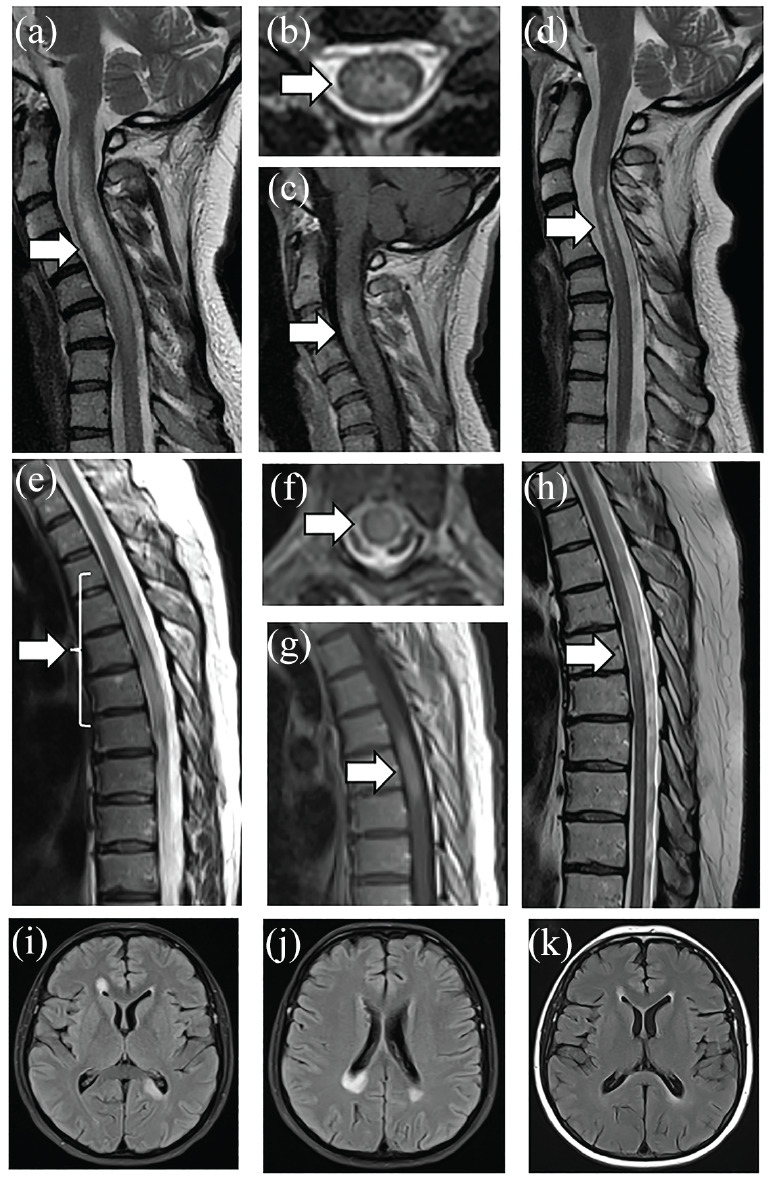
(a to d) A 41-year-old women ultimately diagnosed with AQP4-IgG NMOSD presented with neck pain and left arm weakness with rapid progression more than 2 weeks to quadriplegia and breathing difficulties. Cervical MRI showed longitudinally extensive lesion on T2-weighted imaging with cord swelling (a), central location on axial images (b), and ring-like contrast enhancement (c). The patient was treated with intravenous methylprednisolone followed by plasma exchange with improvement. Follow-up imaging 9 months later showed residual T2-weighted hyperintensity in the central portion of the cord (d). (e to k) A 45-year-old women presented with numbness and weakness in the left leg progressing more than 3 weeks to severe paraplegia with double incontinence and Th6 sensory level despite intravenous methylprednisolone. Spinal cord MRI showed a longitudinally extensive lesion spanning the cord continuously from Th3 to Th7 with mild cord swelling (e). The lesion was located centrally (f) and partially contrast-enhancing (g). Brain MRI showed large poorly demarcated periventricular lesions located in the corpus callosum (i, j). Patient’s serum was negative for both AQP4- and MOG-IgG on fixed cell-based assays locally. The patient was treated with plasma exchange with partial recovery but remained wheelchair-bound. Follow-up imaging of the spinal cord performed 9 months after the onset of symptoms showed partial resolution of LETM lesion (h) and absence of contrast enhancement (not shown). Follow-up brain MRI showed almost complete resolution of brain lesions and absence of new lesions (k). Repeat antibody testing, including live cell-based assays performed in Oxford, remained negative for both AQP4 and MOG antibodies. The patient was diagnosed with seronegative NMOSD and started on rituximab. Both patients consented for their images and clinical summaries to be included in the article (Department of Neurology, Stroke and Neurological Rehabilitation, Wolski Hospital, Warsaw, Poland).
